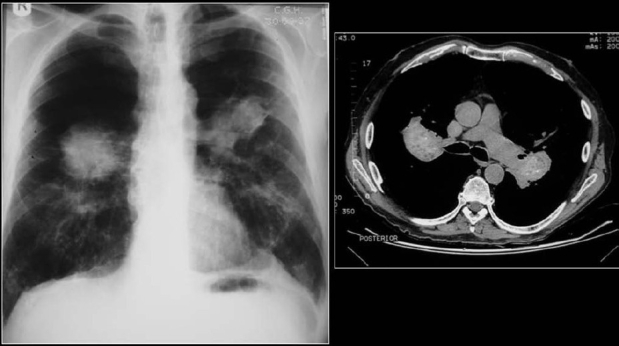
Chest radiograph and an axial CT scan shows calcification PMF in a coal miners lung
